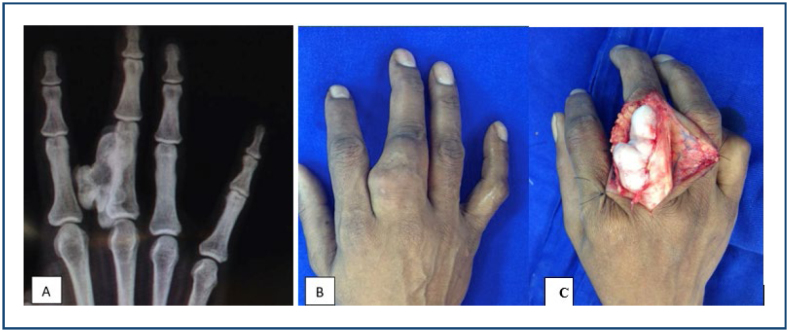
(A) X-ray AP view showing massive osteochondroma on the proximal phalanx of the third finger of the right hand. (B) Clinical image. (C) Exposure of the osteochondroma, with the hyaline cartilage layer being observed.
